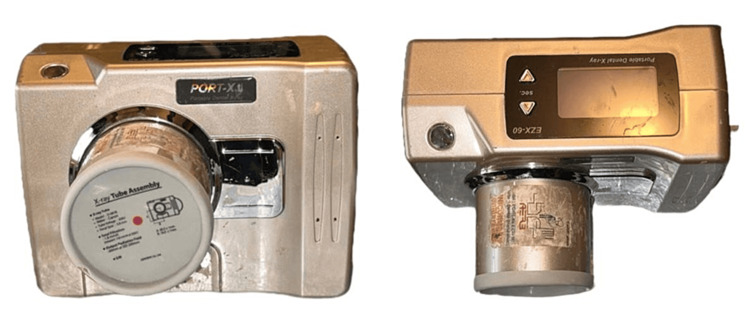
Genoray Port X II handheld dental X-ray unit
